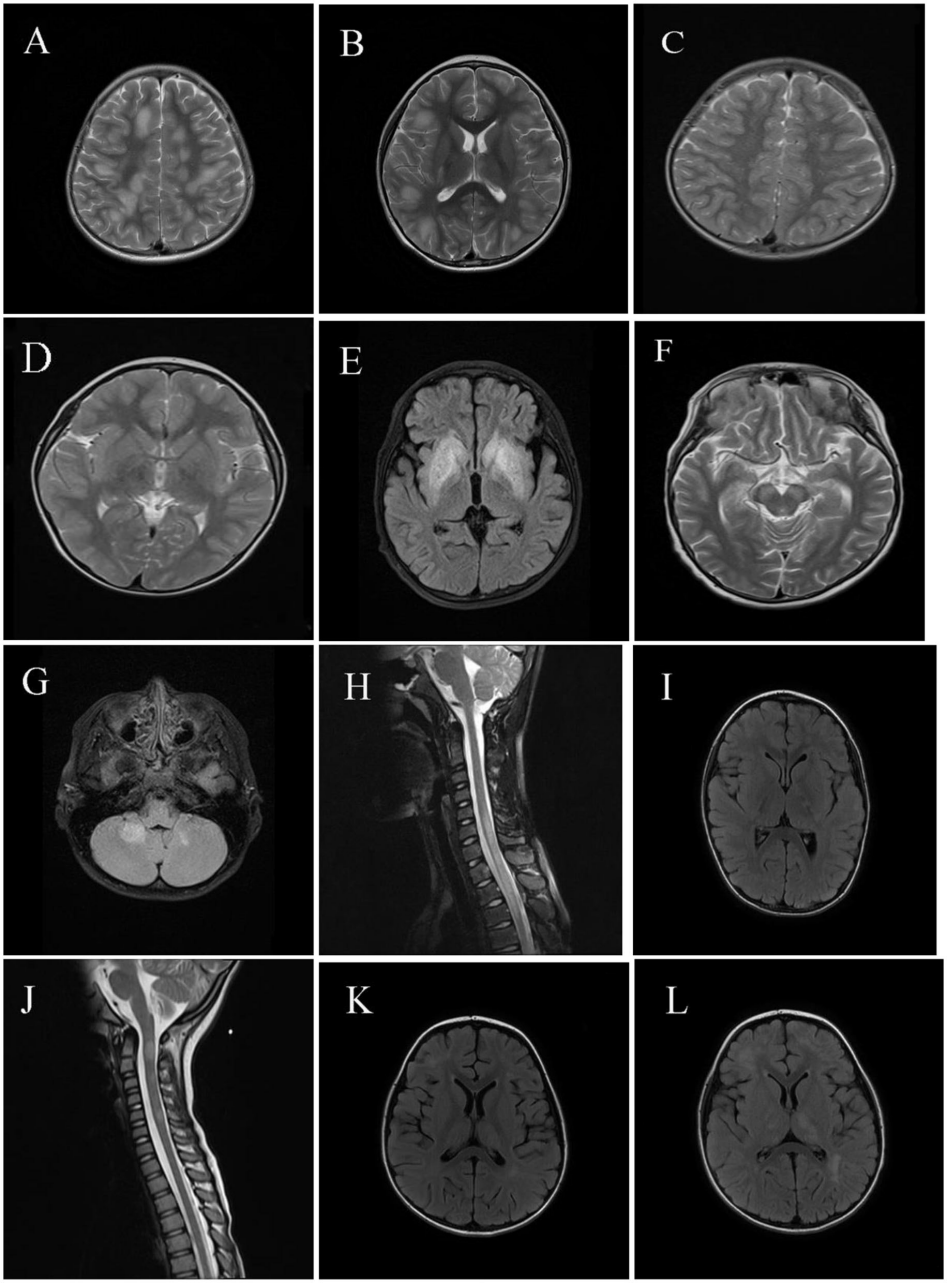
Magnetic resonance imaging (MRI) results of pediatric acute disseminating encephalomyelitis (ADEM) without anti-myelin oligodendrocyte glycoprotein antibodies (MOG-abs). (A–D) Cerebral MRI of a 3-year-old patient with ADEM but without MOG-abs who presented with frequent seizures and coma, revealed widespread, blurred, and large lesions involving supratentorial of both hemispheres and basal ganglia lesions [(A,B); axial-T2] similar to the typical cerebral MRI of patients with ADEM and MOG-abs. Six weeks later, a repeat cerebral MRI showed near-complete disappearance of the abnormal lesions of both supratentorial and basal ganglia [(C,D); axial-T2]. (E,F) Cerebral MRI of a 7-year-old patient with ADEM and lacking MOG-abs, who presented with fever and frequent seizures and had coma, revealed mainly large basal ganglia lesions [(E); axial-T2] and brainstem lesions [(F); axial-T2]; laboratory examinations were performed to exclude other possible diagnoses such as acute poisoning and metabolic encephalopathy; the patient progressively improved within 1 year after immunotherapy and achieved full recovery 1 year after immunotherapy. (G,H) Cerebral and spinal MRI of a 10-year-old patient with ADEM and lacking MOG-abs, who presented with headache, drowsiness, eye pain, fever, and visual dysfunction, showed large and blurred lesions of the cerebellum [(G); axial-T2], LETM of cervical and thoracic spinal cord [(H); sagittal-T2], and supratentorial lesions of the right hemisphere (not shown), and orbital MRI revealed a normal optic nerve (not shown). (I,J) Cerebral and spinal MRI of a 4-year-old patient with ADEM and lacking MOG-abs, who presented with fever, vomiting, urinary incontinence, and disturbance of consciousness, revealed small and blurred lesions of supratentorial, basal ganglia, corpus callosum lesions [(I); axial-T2] and circular or ovoid lesions of the cervical spinal cord [(J); sagittal-T2]. (K,L) Cerebral MRI of a 4-year-old patient with ADEM and lacking MOG-abs, who presented with fever, headache, vomiting, seizures, and disturbance in consciousness, revealed small and hazy lesions of supratentorial, thalamus/basal ganglia [(K); axial-T2]; 4 weeks later, lesions of the supratentorial and thalamus/basal ganglia enlarged even after immunotherapy, but the patient progressively achieved clinical improvement [(L); axial-T2].
